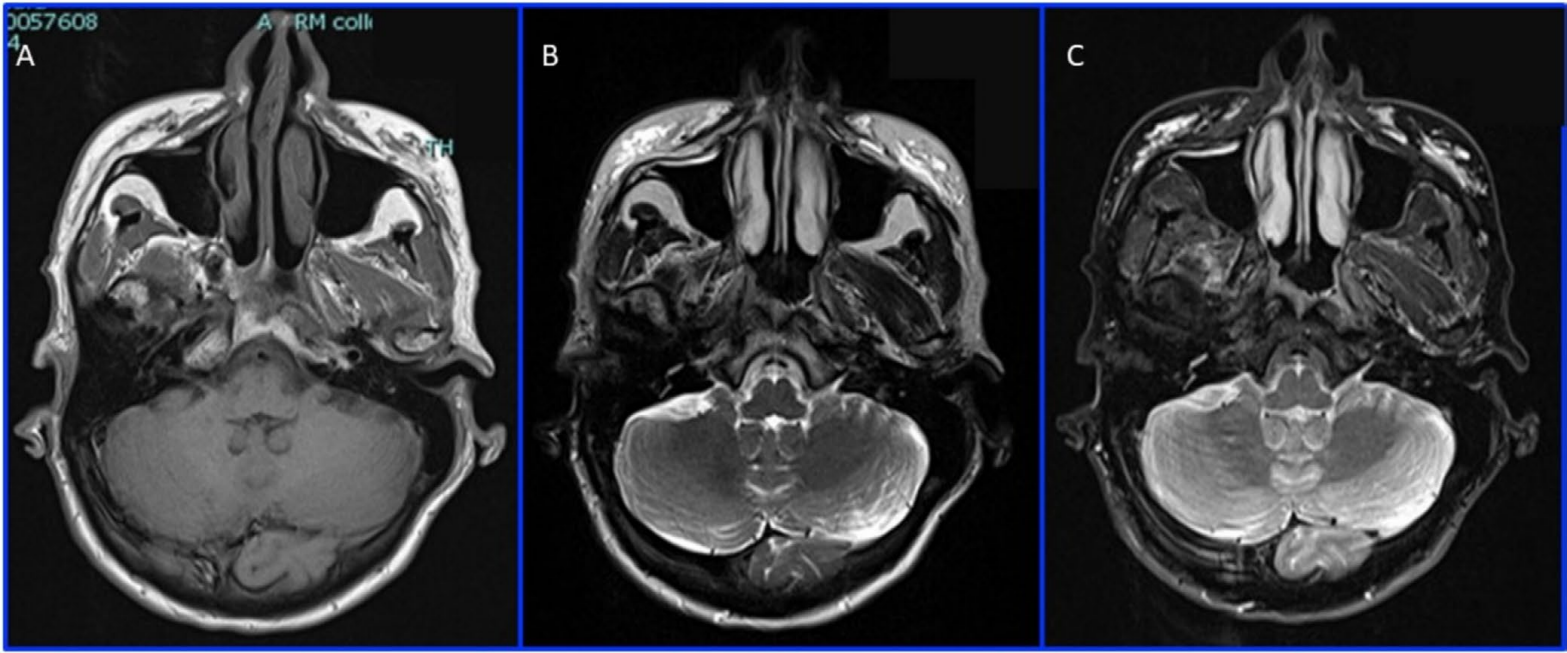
MRI: Axial T1 (A), T2 (B), and T2 TIRM (C) sequence of the III MRI, showing the presence of HA filler in the zygomatic region bilaterally; in (A) we can see the filler as low‐intensity signal areas; in (B) we identify the filler as high‐intensity signal areas; in (C) the filler is represented by very high‐intensity signal areas; we can see how T2 TIRM is the best sequence for highlighting the HA filler.
